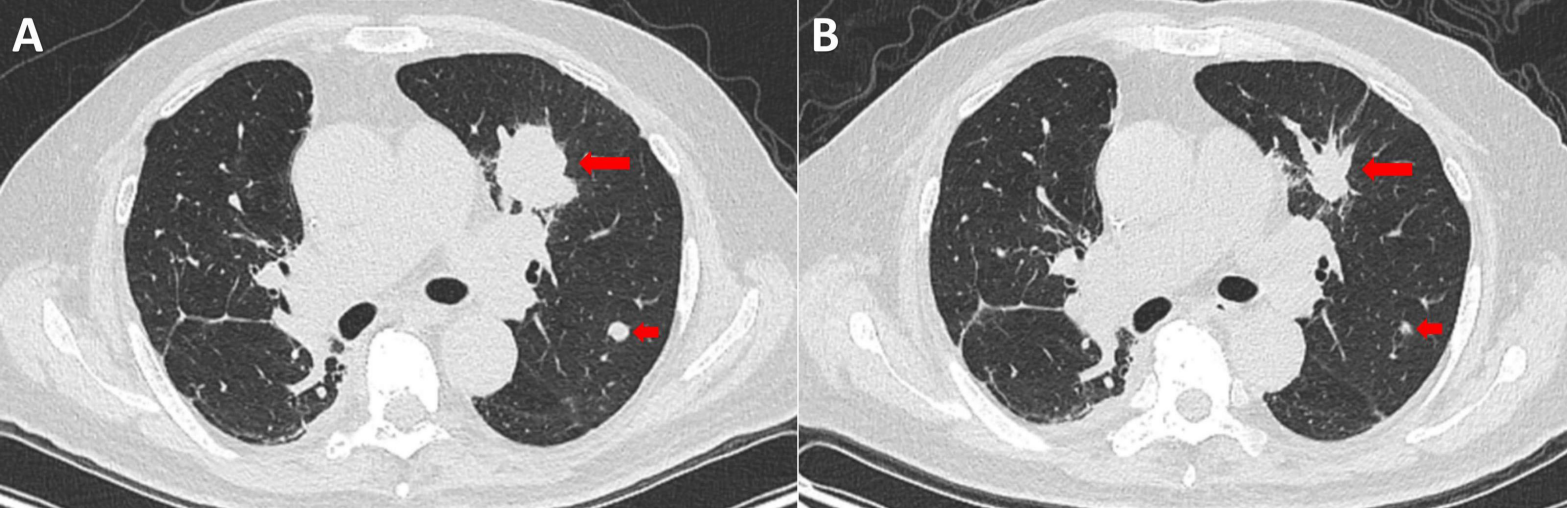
Contrast-enhanced chest CT images before and after treatment initiation. (A) Pre-treatment scan (October 23, 2025) shows multiple tumor lesions (red arrow) in the left upper lobe. (B) Scan obtained during the CRS episode following amivantamab-chemotherapy (November 5, 2025) demonstrates marked reduction in the size of the multiple lesions (red arrow).
